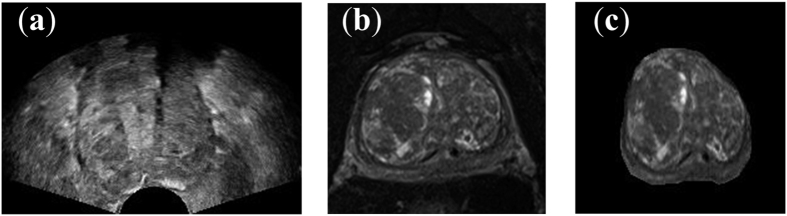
Example slices: (a) the TRUS image, (b) the MR image, (c) the registered MR image using our deformation model.
